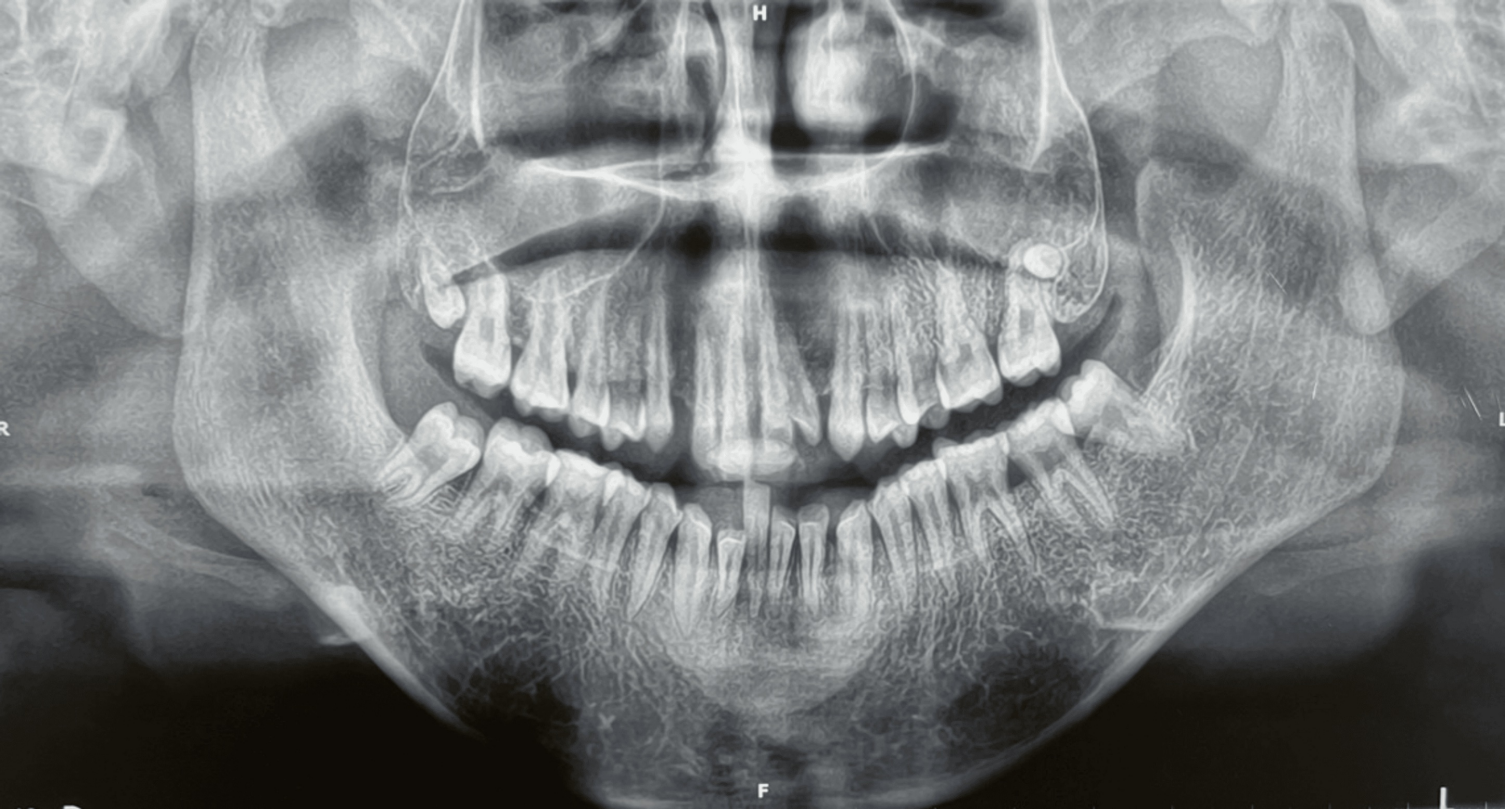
Pre-operative orthopantomogram (OPG) panoramic radiograph showing the presence of all permanent teeth along with developing upper third molars and no evidence of alveolar bone loss.
